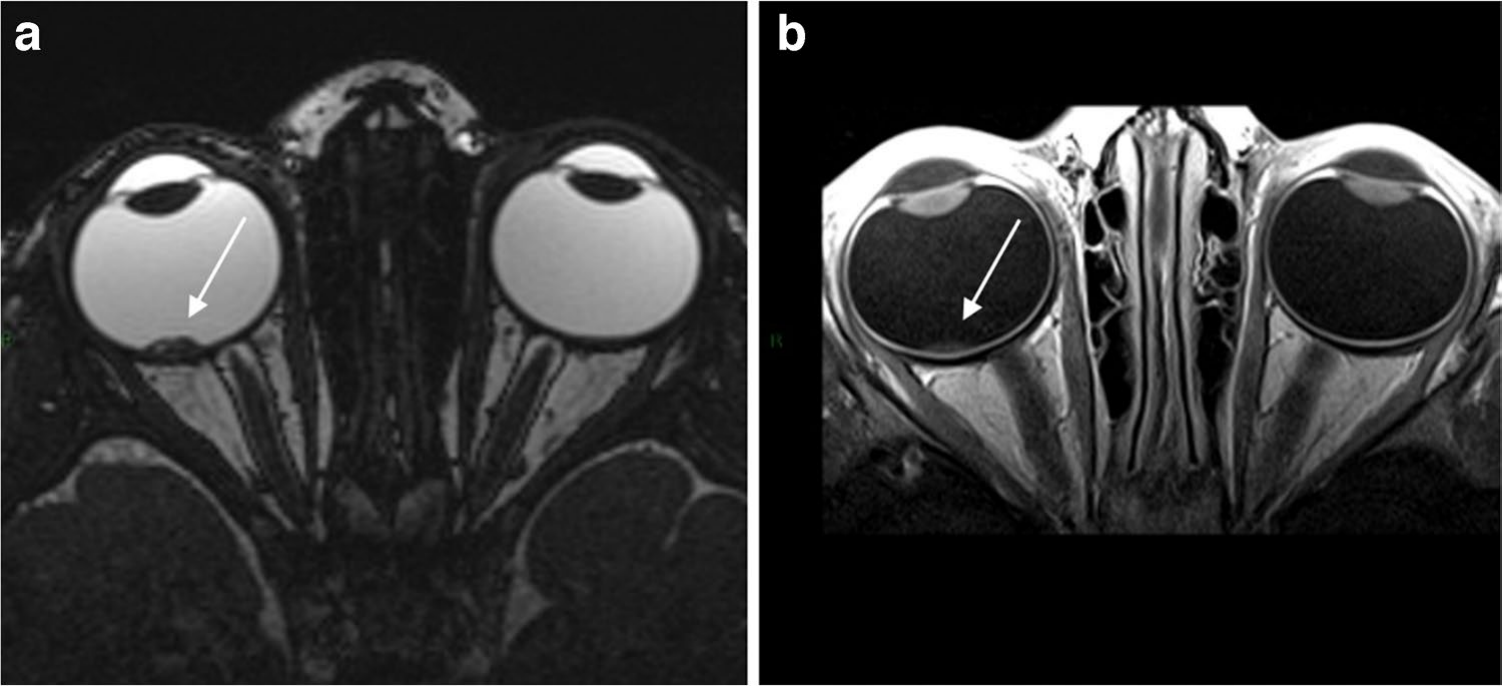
MRI of a 19-month-old girl at the time of imaging, diagnosed with retinocytoma. Axial T2-weighted (a) and post-contrast T1-weighted (b) images show (*arrow*) a slightly enhancing macular mass. *MRI*, magnetic resonance imaging
